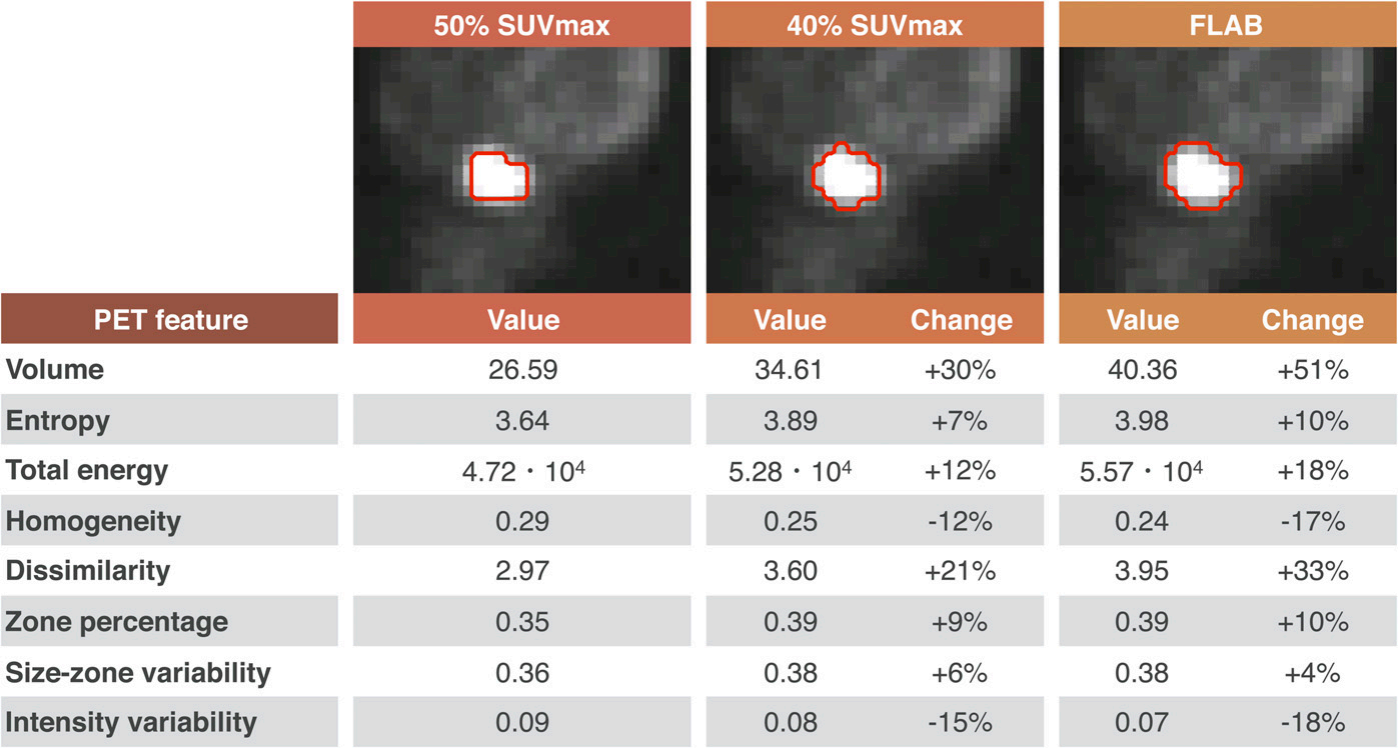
Positron emission tomography (PET) radiomic features^14^ and their dependency on the delineation method in oesophageal cancer: the 50% maximum standardized uptake value (SUV_max_) delineation is used as reference. Fuzzy locally adaptive Bayesian (FLAB) delineation was implemented as described previously.^51^
